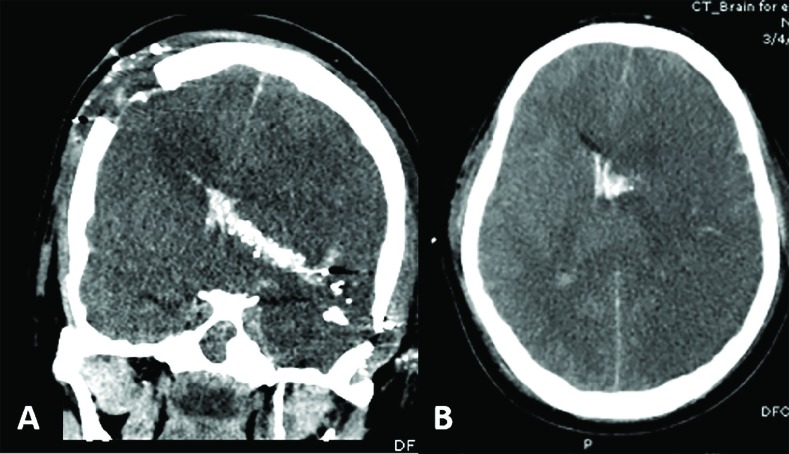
Perforating injury. (A) Coronal plane CT of the brain shows bullets pass at the right parietal area and cross through multilobar involvement. Finally, the bullets exit through at left temporal area. (B). Axial CT of the brain showed diffuse brain swelling, intraventricular hemorrhage, and subarachnoid hemorrhage
